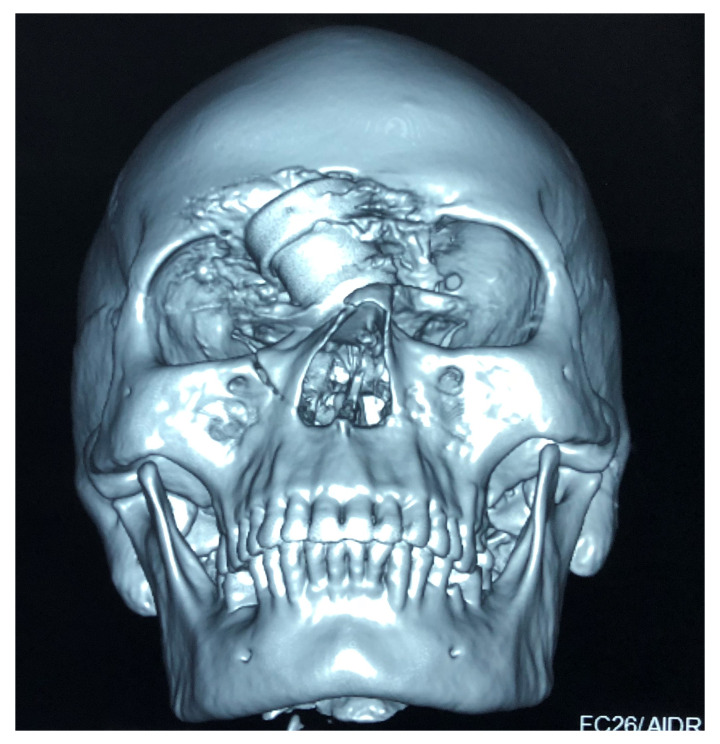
Plain Computed Tomogram of the face and skull (3D reconstruction) showing the foreign body and its relation to the right orbit.
